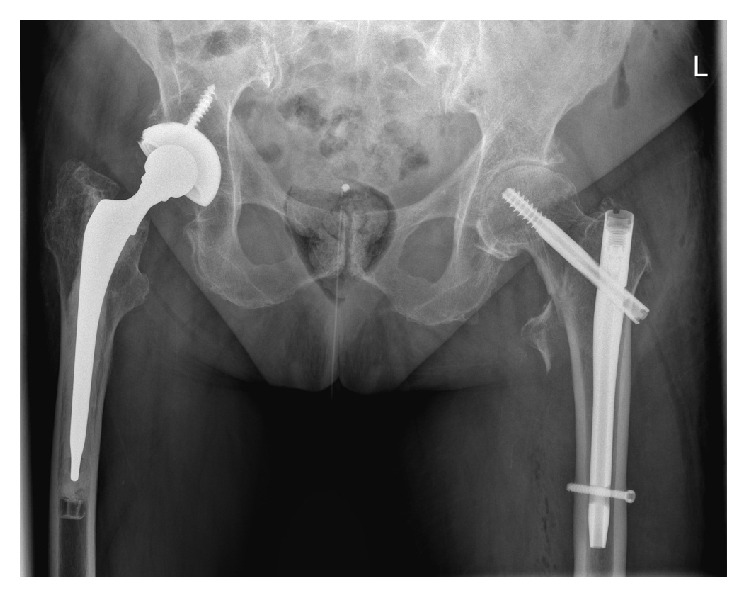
Anterior-posterior postoperative X-ray showing good positioning of the intramedullary Gamma nail in the right femur, with a tip-apex distance below 25 mm combined (AP+ axial).
